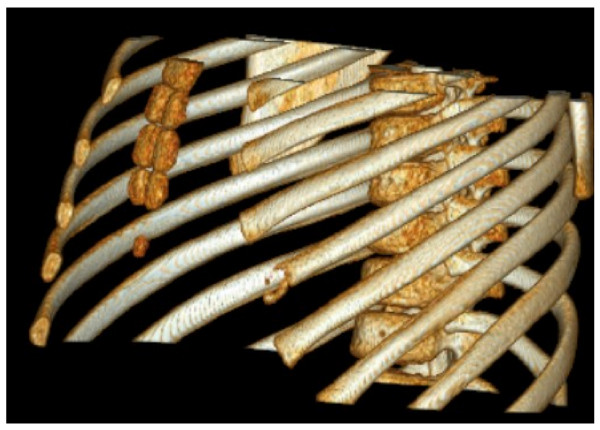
Sibling B - CT Chest showing osteolysis of the left 6^th ^rib, aged 10 1/2.
